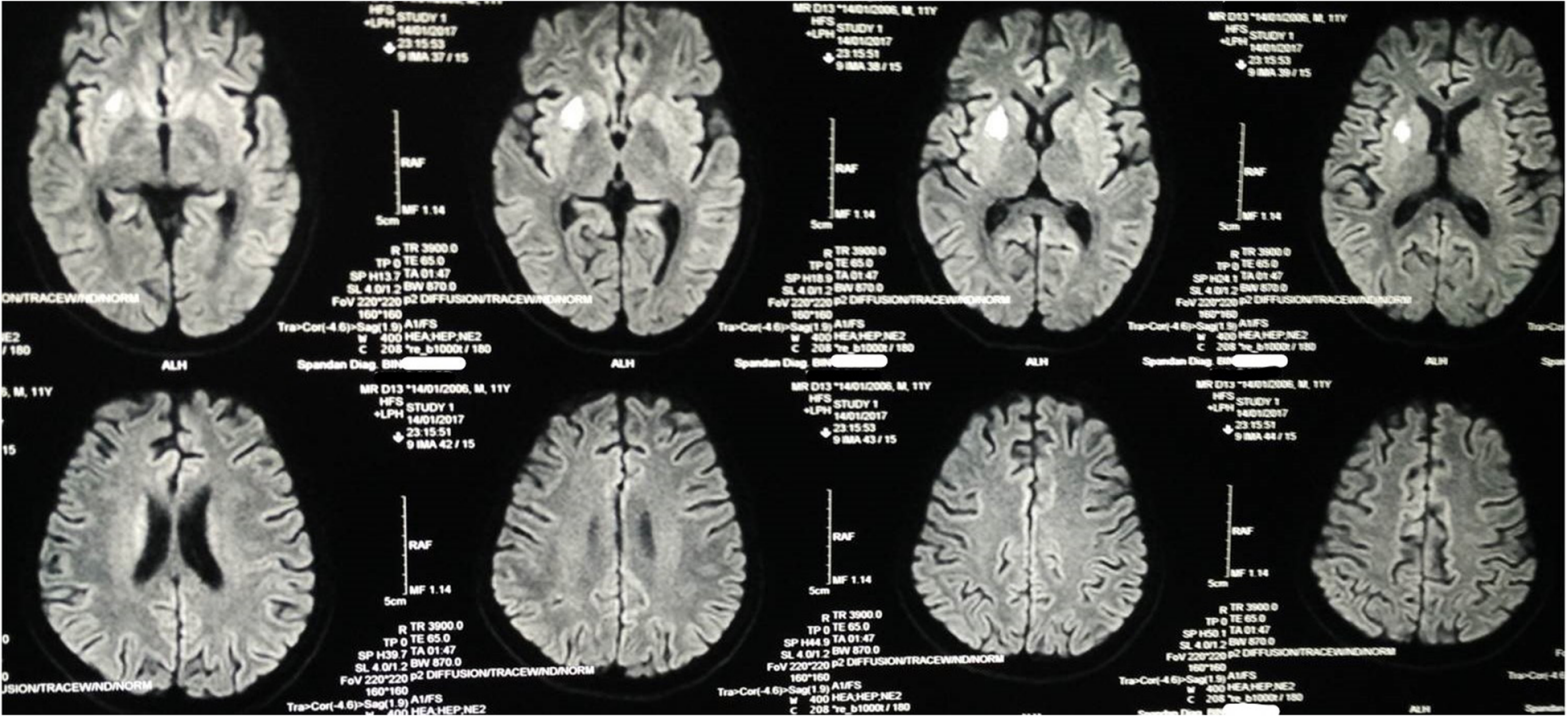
Magnetic resonance imaging of the brain (diffusion-weighted sequence) shows a small area of diffusion restriction in the capsule-ganglionic area
